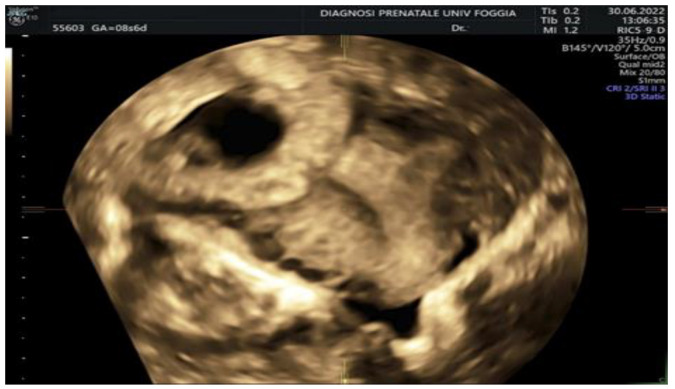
3D transvaginal ultrasound scan performed on day 15 showing the interstitial pregnancy.
